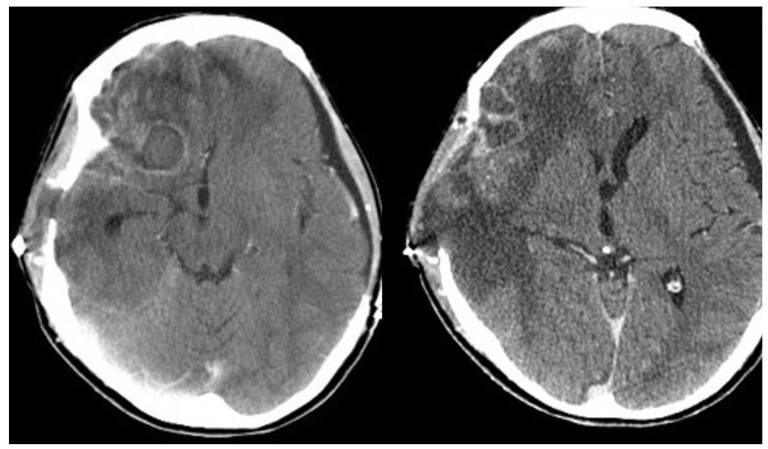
Axial contrast-enhanced CT images following bilateral craniectomy performed 2 weeks ago. The exam displayed postoperative changes more evidently in the right cerebral hemisphere, where concurrent peripherally enhancing lesions were also demonstrated, primarily suspicious of representing abscesses.
